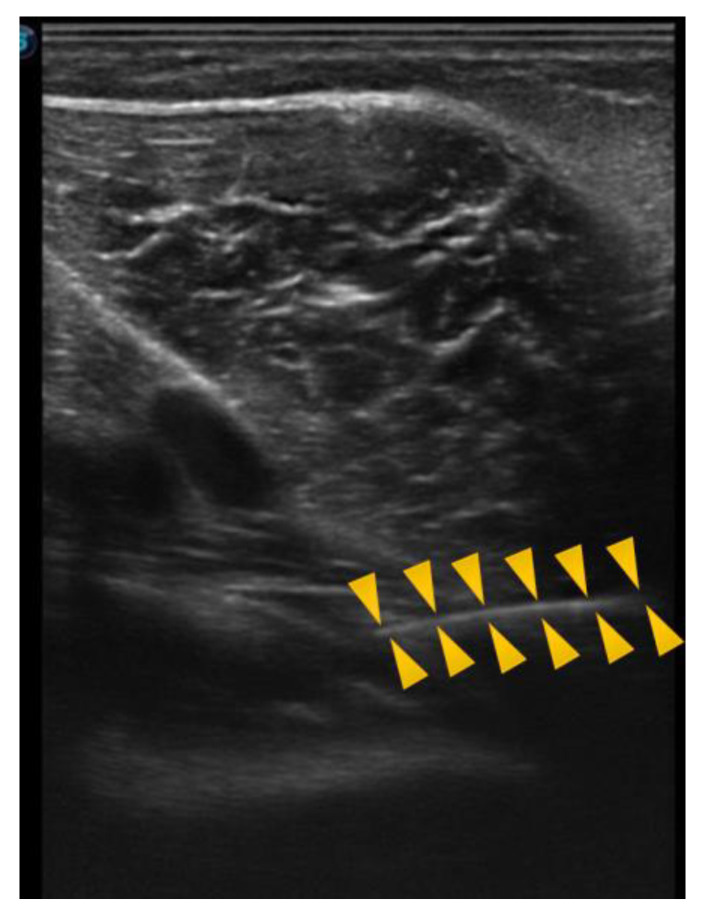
Ultrasound of the popliteal puncture in the internal superior region. Head arrows define the needle line.
